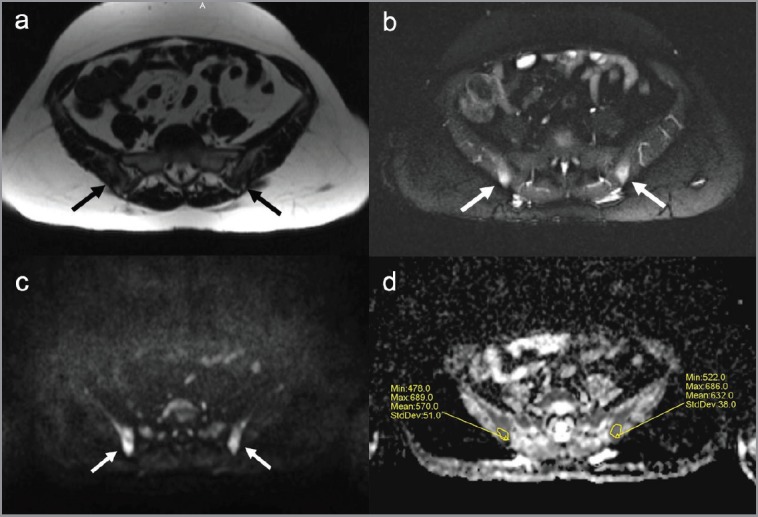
Pelvic bone lesions on diffusion whole-body MRI. Diffusion whole-body MRI showed ill-defined abnormalities in the iliac bones (arrows). The lesions presented low signal on T1-weighted images (a) high signal on STIR images (b) and on diffusion-weighted images with b-value of 900 s/mm^2^ (c), impeded diffusion of water molecules in ADC map with ADC values ranging from 478 to 686 µm^2^/s (d).
